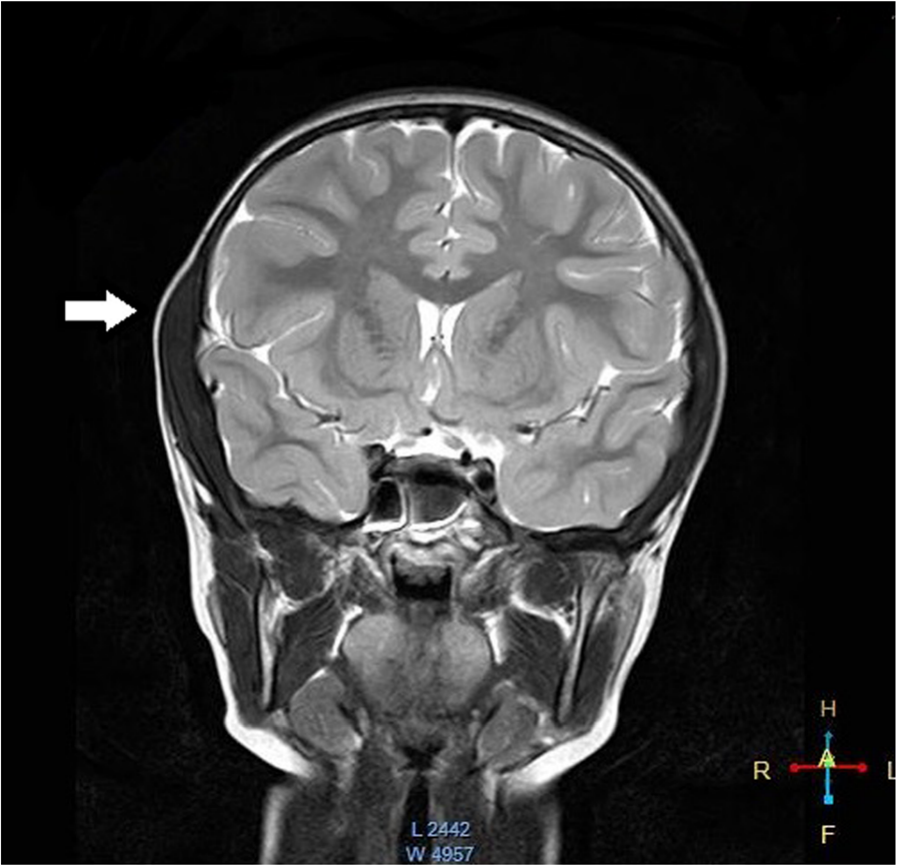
Isolated right temporalis muscle hypertrophy on a coronal MRI image. T2W image of the cMRI study demonstrating enlarged right temporalis muscle on a coronal section without abnormal contrast enhancement. White arrow head indicate enlarged right temporalis muscle
